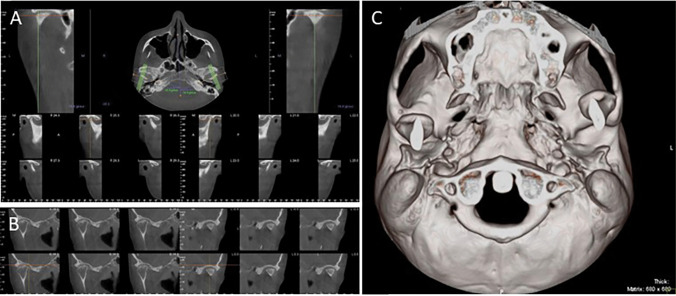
A TMD diagnosis documented by DCIM images from CBCT evaluation of a 37-year-old female (sample no. 2). This corresponded to coronal images between horizontal (in the upper) and sagittal slices of the right TMJ (R24.3 to R29.3) and the left TMJ (L20.0 to L25.0) identifying the following anatomic and functional deficits: severe flattening of the condyle and osteophytes, with a reduced joint space and a general increase in density to the glenoid fossa. B TMD diagnosis documented by DICOM images from CBCT evaluation of a 57-year-old male (sample no. 45). This corresponded to sagittal images of the right TMJ (R18.5 to 19.6) and left TMJ (L0.0 to 0.8), identifying the following anatomic and functional deficits: severe flattening of the left condyle and osteophytes, reduced joint space, namely, in the right with erosion of the cortex and increased density to the glenoid fossa. C TMD diagnosis was documented by tridimensional reconstruction of DICOM images from CBCT of the open mouth of a 42-year-old female (sample no. 66). This corresponds to an abnormal position of the left condyle following a functional deficit in extreme open-mouth activity
